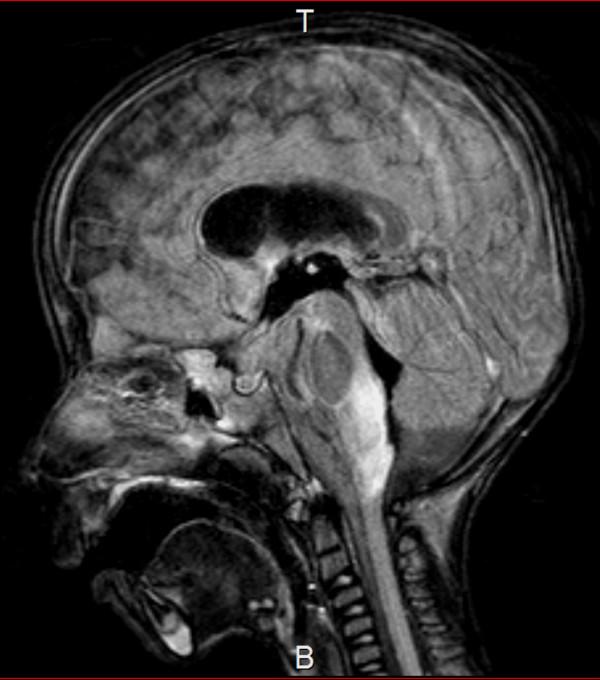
Magnetic resonance imaging at 4 years. a T2-weighted image shows significant volume loss in bilateral white matter and deep gray matter as a cause of enlargement of the anterior horn of the lateral ventricle. b1,2 T1-weighted image and T2-weighted image show the periventricular rim around the posterior horn of the lateral ventricle. c Abnormal signals in the medulla oblongata become apparent in T2-weighted image.
